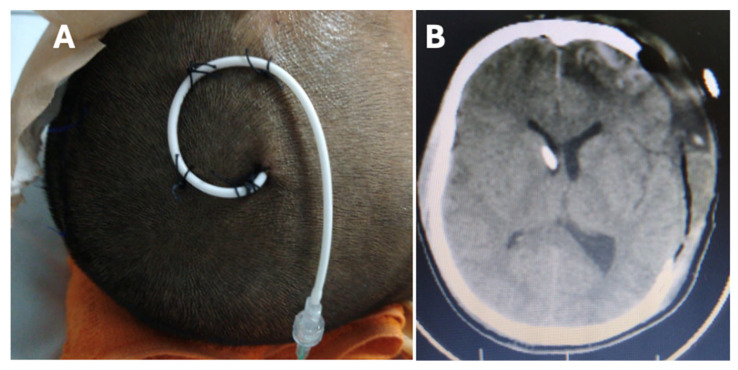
Panel (A) shows the external ventricular drain (EVD) tunneled subcutaneously and secured to the scalp with sutures. The catheter exit site is clean, and the system is connected to a closed sterile drainage circuit for intracranial pressure monitoring and cerebrospinal fluid diversion. Panel (B) shows a CT scan demonstrating the trajectory of the EVD inserted through a frontal approach (Kocher’s point). The catheter follows an appropriate intraparenchymal course, and the tip is correctly positioned within the frontal horn of the lateral ventricle, confirming adequate placement.
